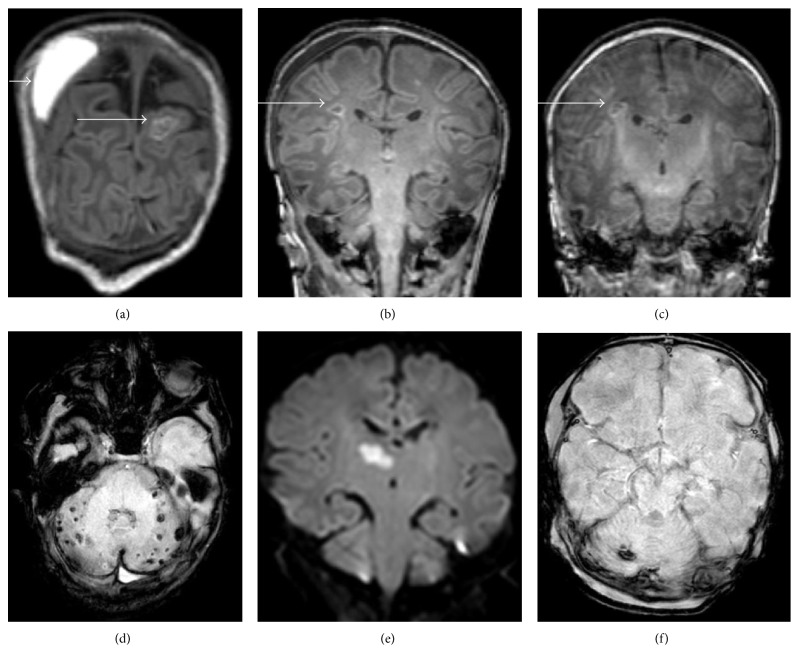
Examples of parenchymal and nonparenchymal brain injury. (a) Coronal T1-weighted image: cortical infarction and subdural haemorrhage; (b) coronal T1-weighted image: white matter lesion; (c) coronal T1-weighted image: white matter lesion; (d) susceptibility weighted image: multiple punctate cerebellar lesions; (e) diffusion-weighted image: thalamic infarction; (f) susceptibility weighted image: cerebellar haemorrhage.
